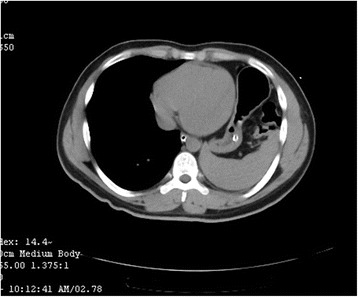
CT scan chest which revealed defect in the left diaphragm posteriorly, consistent with the diagnosis of diaphragmatic rupture with resultant herniation of the stomach, splenic flexure of the colon, spleen, omental fat, descending colon, left-sided transverse colon, bowel, mesentery and in the left hemi-thorax
